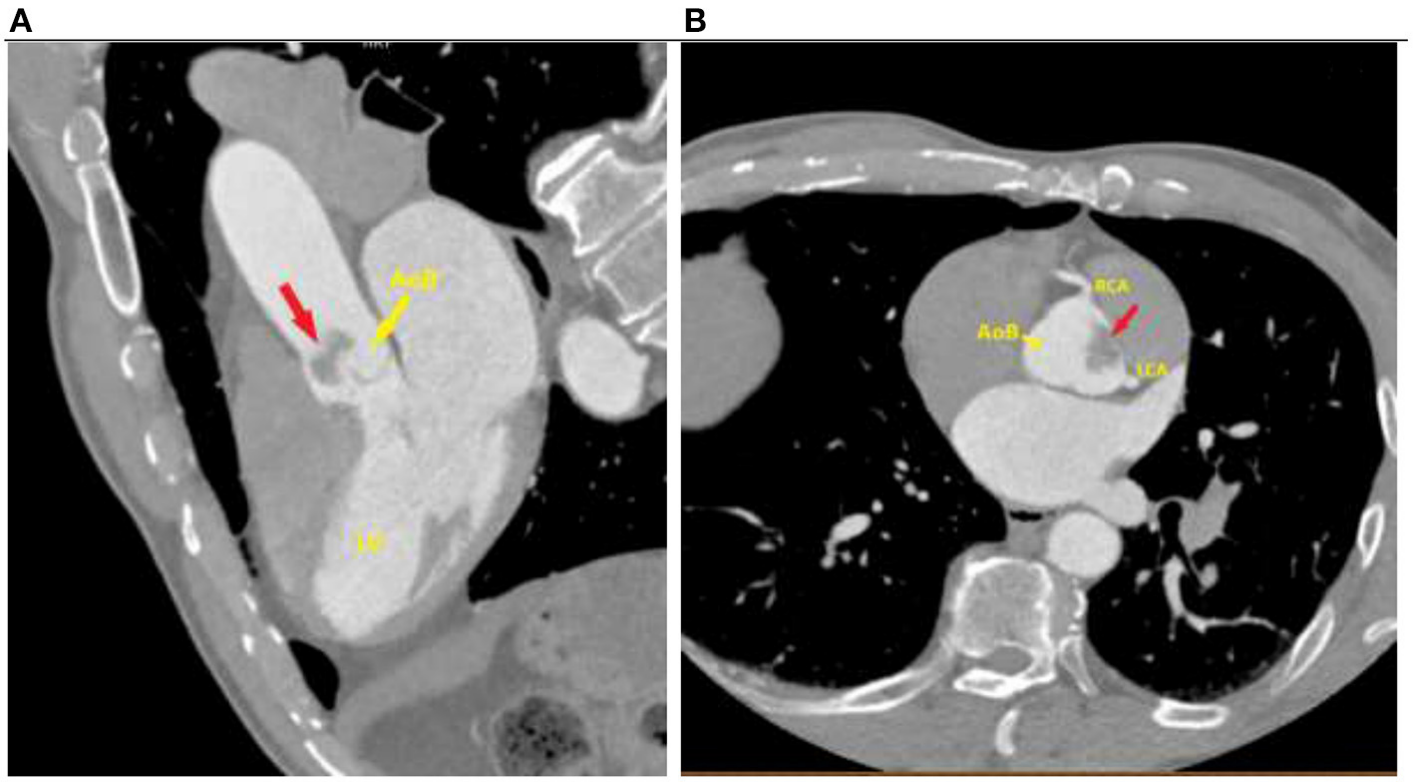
Computed tomography of the chest: (A) A large fibroelastoma (red arrow) in the aortic bulb. (B) Cross-section showing localization of fibroelastoma (red arrow) right next to the left coronary artery ostium. (AoB) Aortic bulb. (LV) Left ventricle. (LCA) Left coronary artery. (RCA) right coronary artery.
